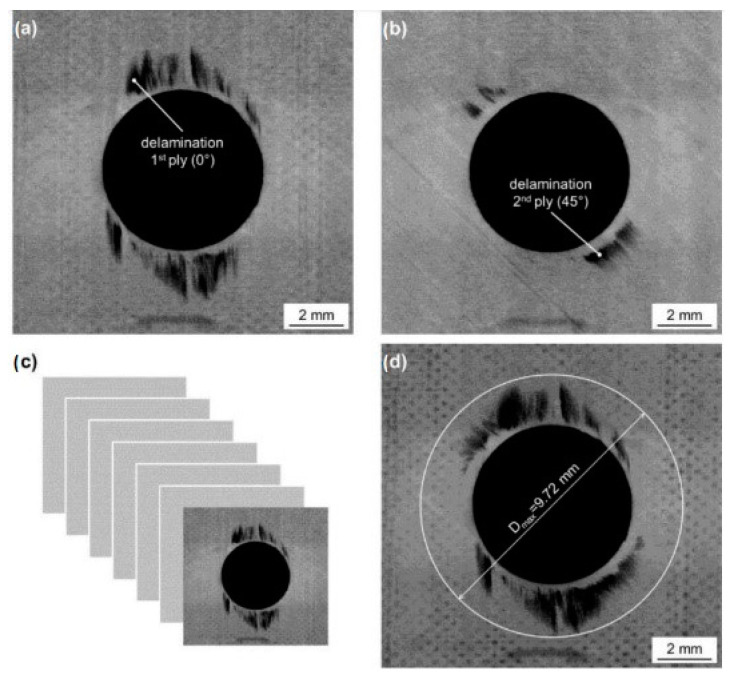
CT images of drilled holes with different levels of delamination to varying drilled hole depths [20].
